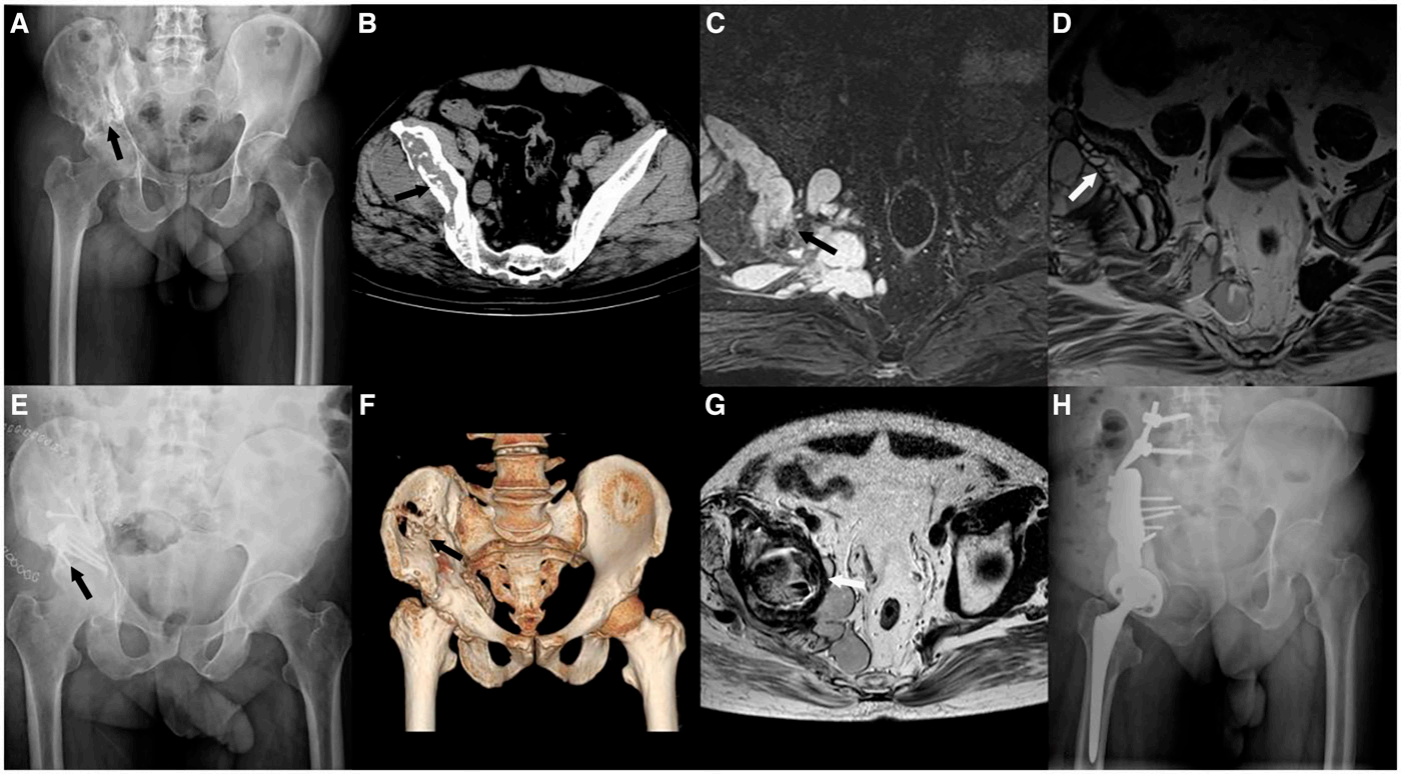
A 46-year-old man presented with right hip/groin pain, swelling, and a painful mass of the right hip. (A) Preoperative anteroposterior X-ray of the pelvis showed osteolytic bone destruction around the right ilium, sacroiliac joint, and acetabulum (black arrow). (B–D) Preoperative computed tomographic scan and magnetic resonance (MR) images showed bone destruction in regions I, II, and IV of the right pelvis and proximal right femur (black arrows and white arrow). (E) Anteroposterior X-ray of the pelvis after debridement with the bone defect filled by bone cement and fixation with a plate internal fixation (black arrow). (F, G) Computed tomographic three-dimensional reconstruction and MR image at postoperative year 2 after the first operation showed multiple areas of bone destruction around the right ilium, acetabulum, and upper femur, indicating signs of recurrence of infection (black arrow and white arrow). (H) Postoperative anteroposterior X-ray of the pelvis showed good alignment of the femoral prosthesis and pelvic prosthesis with stable pelvic reconstruction.
